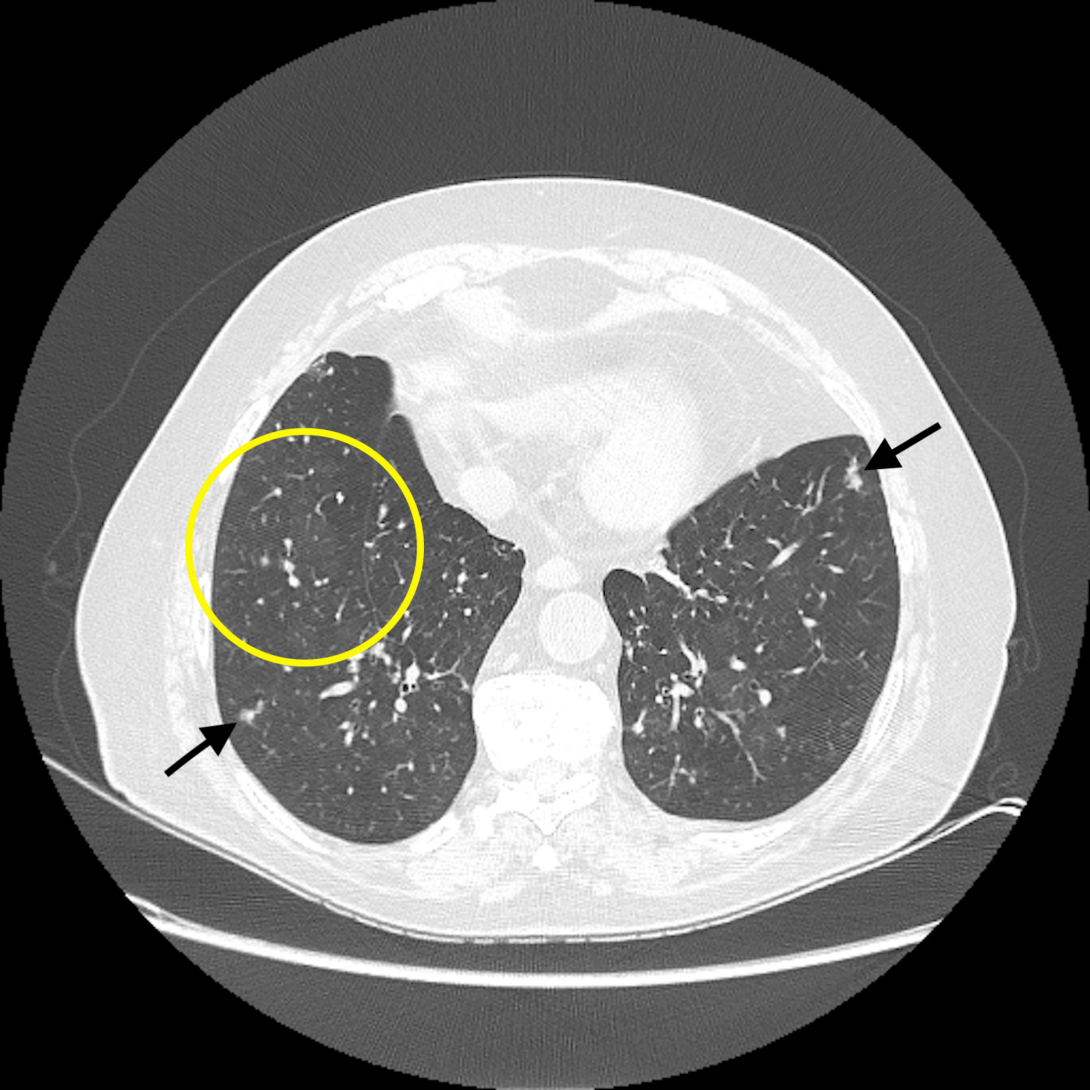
Chest high-resolution computed tomography (HRCT) at reassessment Multiple small nodules (black arrows) and areas of mosaic attenuation (yellow circle).
